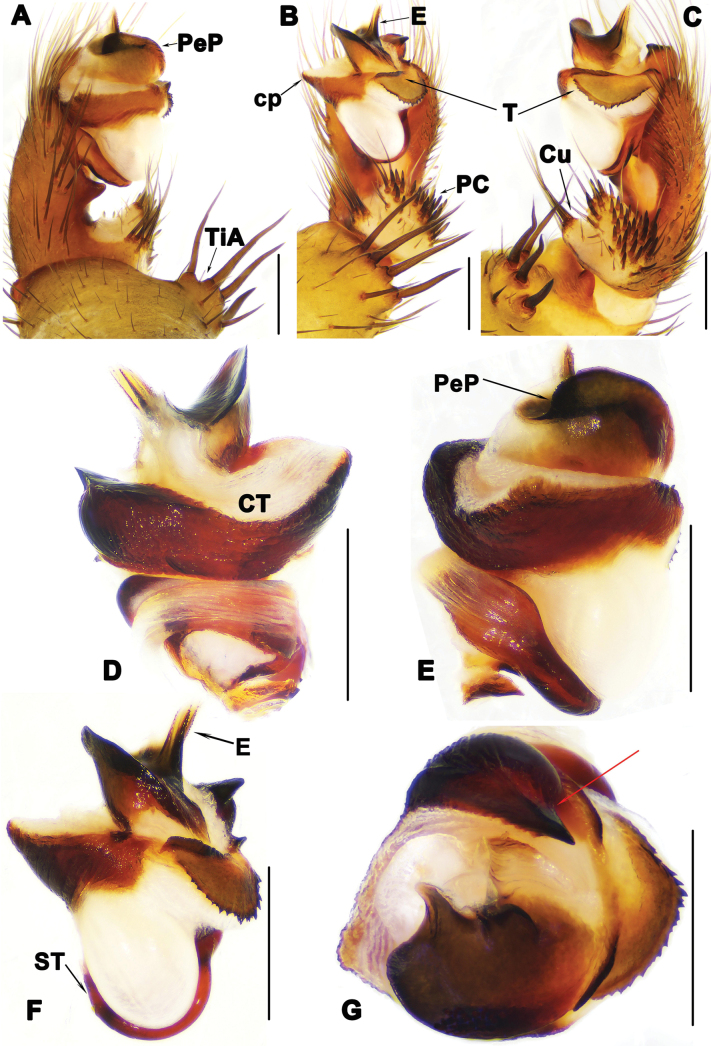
Male palp of *Liphistiusrostratus* sp. nov. A, E prolateral view B ventral view C, F retrolateral view D dorsal view G distal view, red arrow shows beak-like contrategular projection. Abbreviations: CT = contrategulum; cp = contrategular process; Cu = cumulus; E = embolus; PC = paracymbium; PeP = paraembolic plate; ST = subtegulum; T = tegulum; TiA = tibial apophysis. Scale bars: 0.5 mm.
